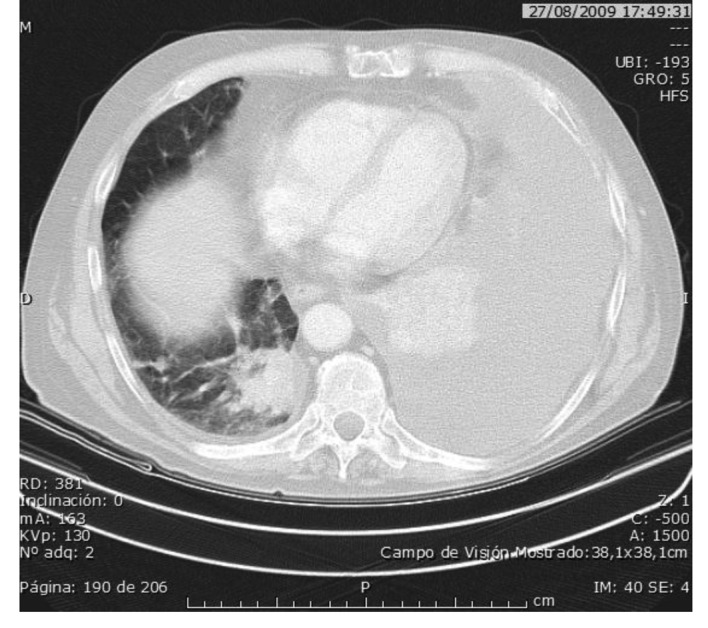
August 2009: The CT scan showed a growth of lung metastases and massive pleural effusion in the left hemithorax.
